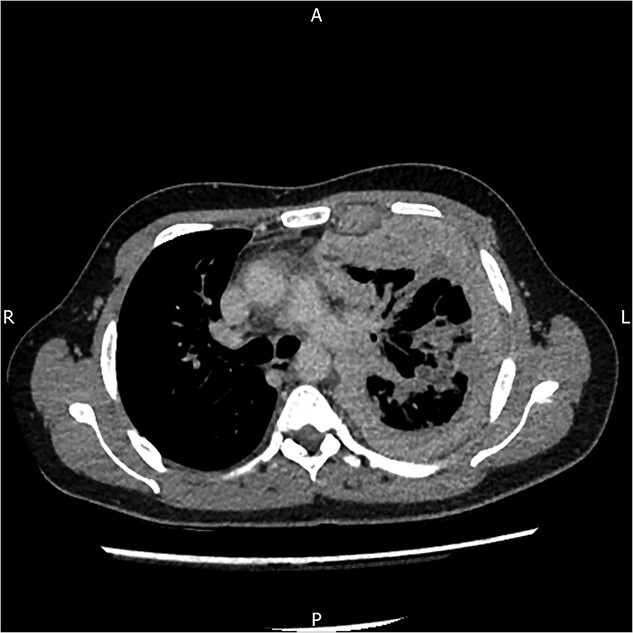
CT scan of the thorax that shows left sided pleural thickening. CT scan of the thorax.
